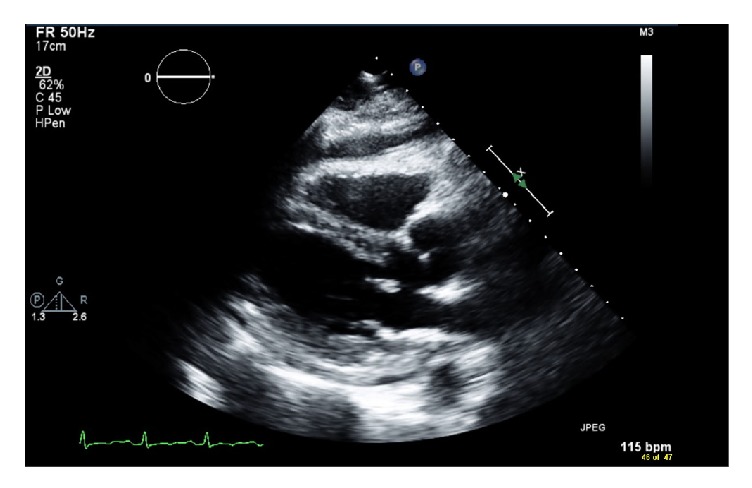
Parasternal long view of the pericardial effusion on transthoracic echocardiogram.
